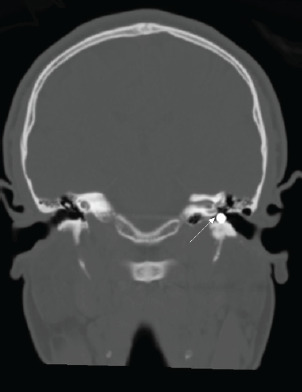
Coronal view of non-contrast computed tomography of the head. White arrow points to metallic foreign body in the patient’s left external auditory canal.
